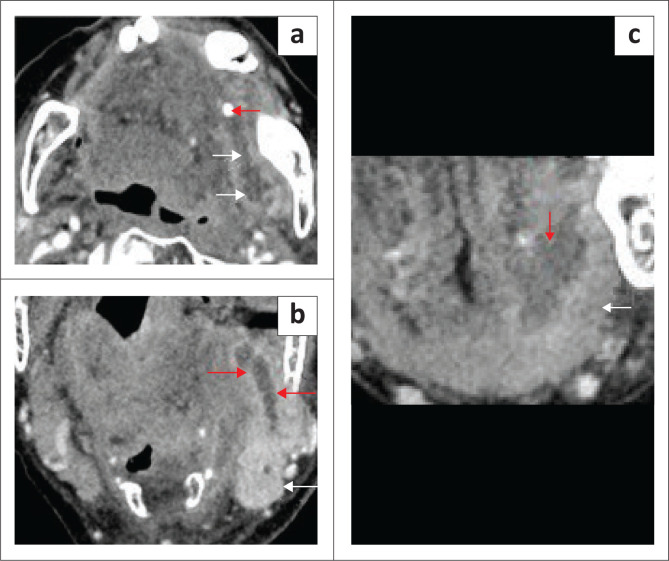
(a, c) Obstructing submandibular duct calculus with adenitis and a floor of the mouth (FOM) abscess. A 98-year-old female patient presented with a large, tense swelling in the left FOM and submandibular region, a swollen tongue and difficult breathing. Contrast-enhanced axial CT neck (a) demonstrated a calcified obstructing calculus at the distal end of the left submandibular duct (red arrow) and a markedly dilated duct (white arrows). The left submandibular gland was enlarged and markedly enhancing because of acute adenitis (b, white arrow and dilated duct, red arrows). There is a left anterior FOM collection (c, red arrow), deep to the left mylohyoid muscle (c, white arrow).
